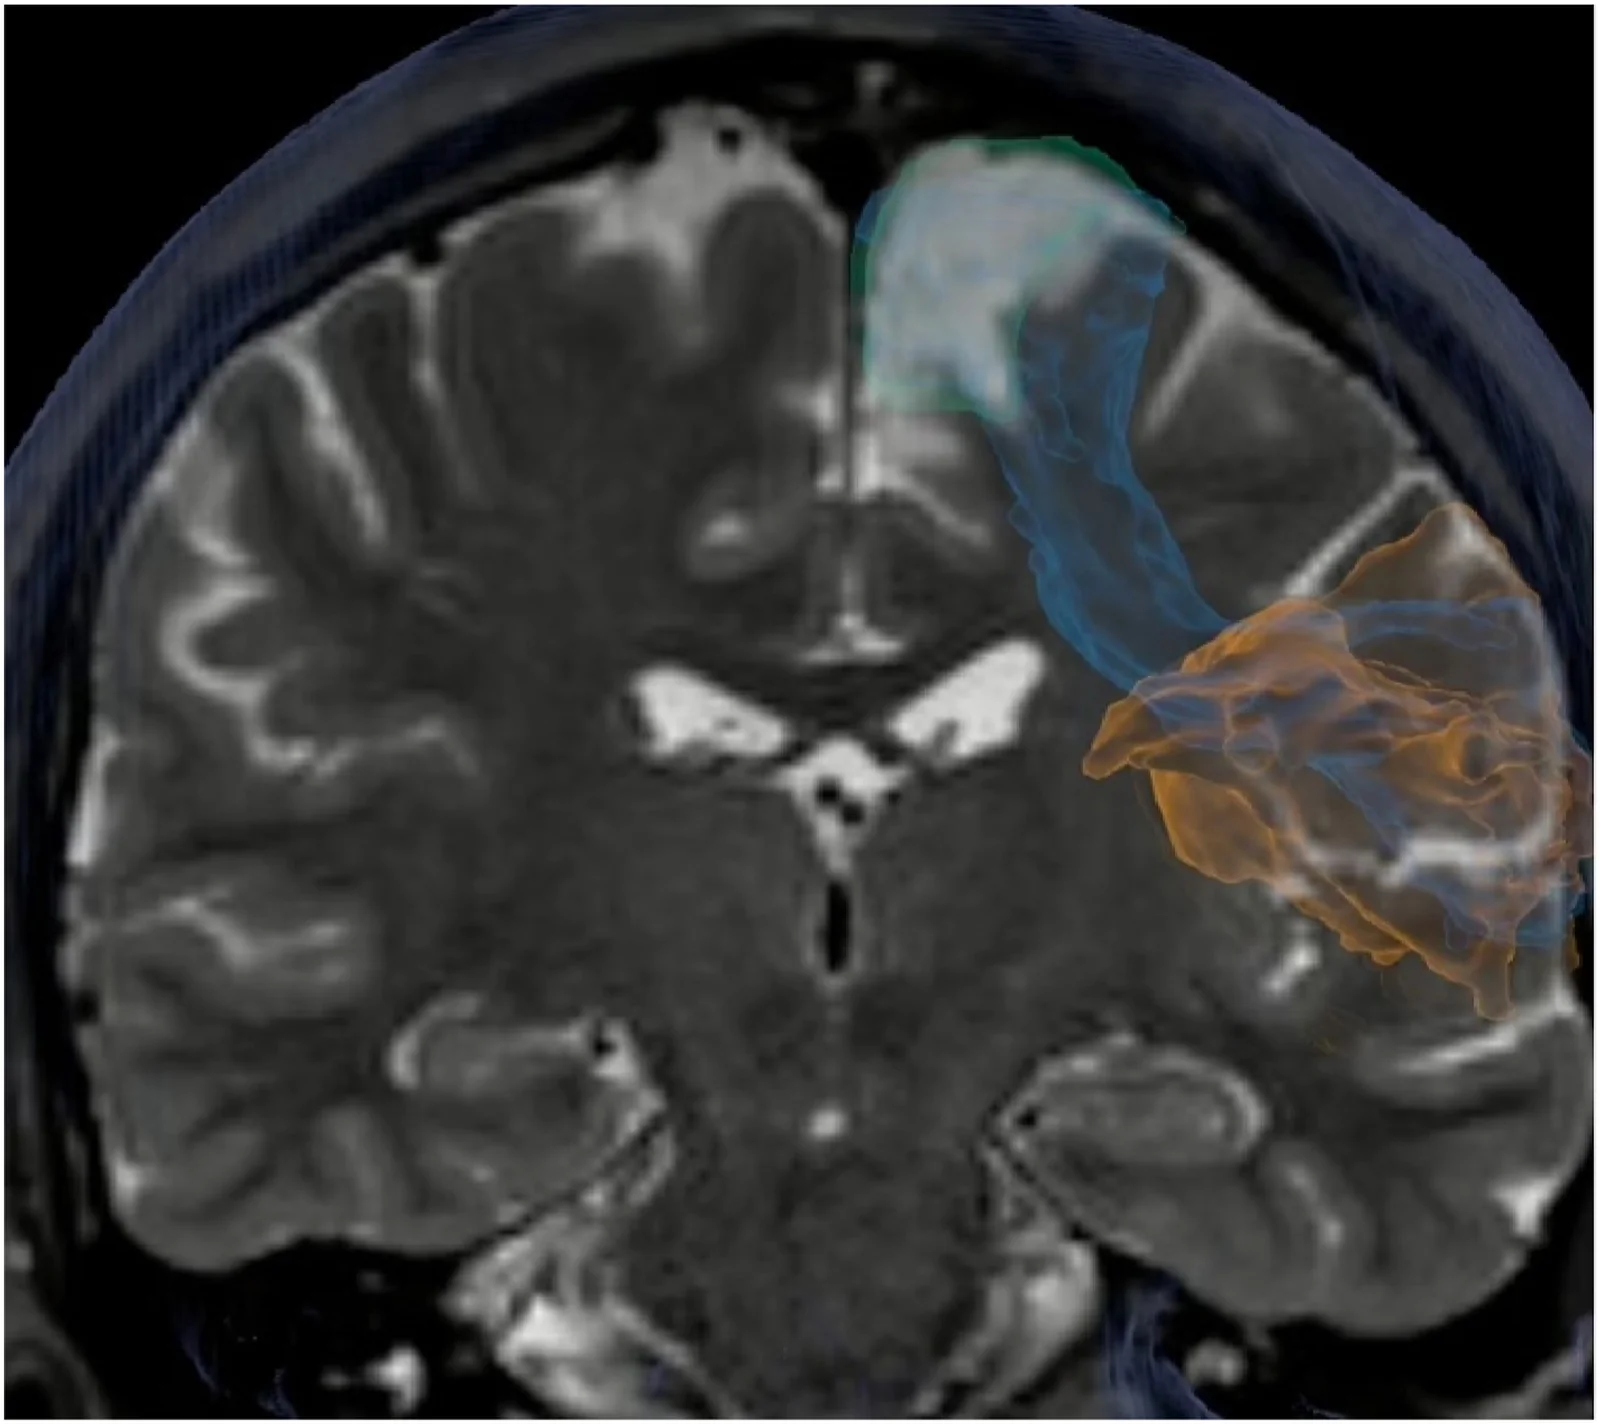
Coronal slide of a T2 postoperative MRI. The resection cavity of the low-grade glioma involving the supplementary motor area region of the superior frontal gyrus is depicted in green. The frontal aslant tract crossing the glioma is visualized in blue, whereas the arcuate fasciculus is shown in orange. DTI fiber-tracking parameters were set to FA = 0.15, length = 50 mm, angulation = 45° as described under methods 2.5.
